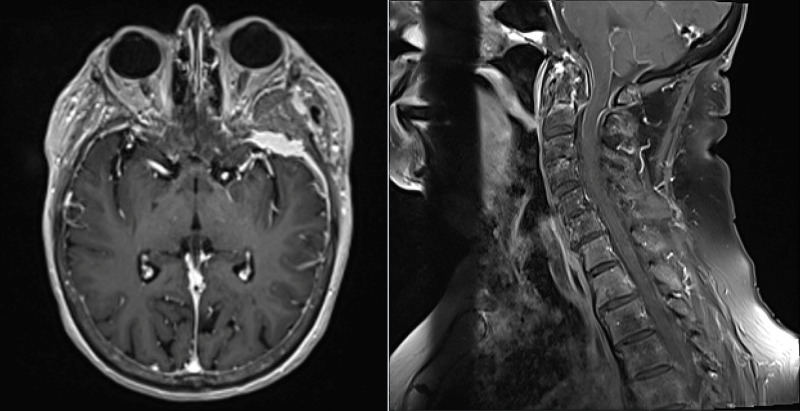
MRI brain and cervical spine with and without contrast. Brain MRI showing a stable appearance of a left temporal meningioma and enhancement along the leptomeninges lining the cerebellum and upper cervical spinal cord. MRI, magnetic resonance imaging
